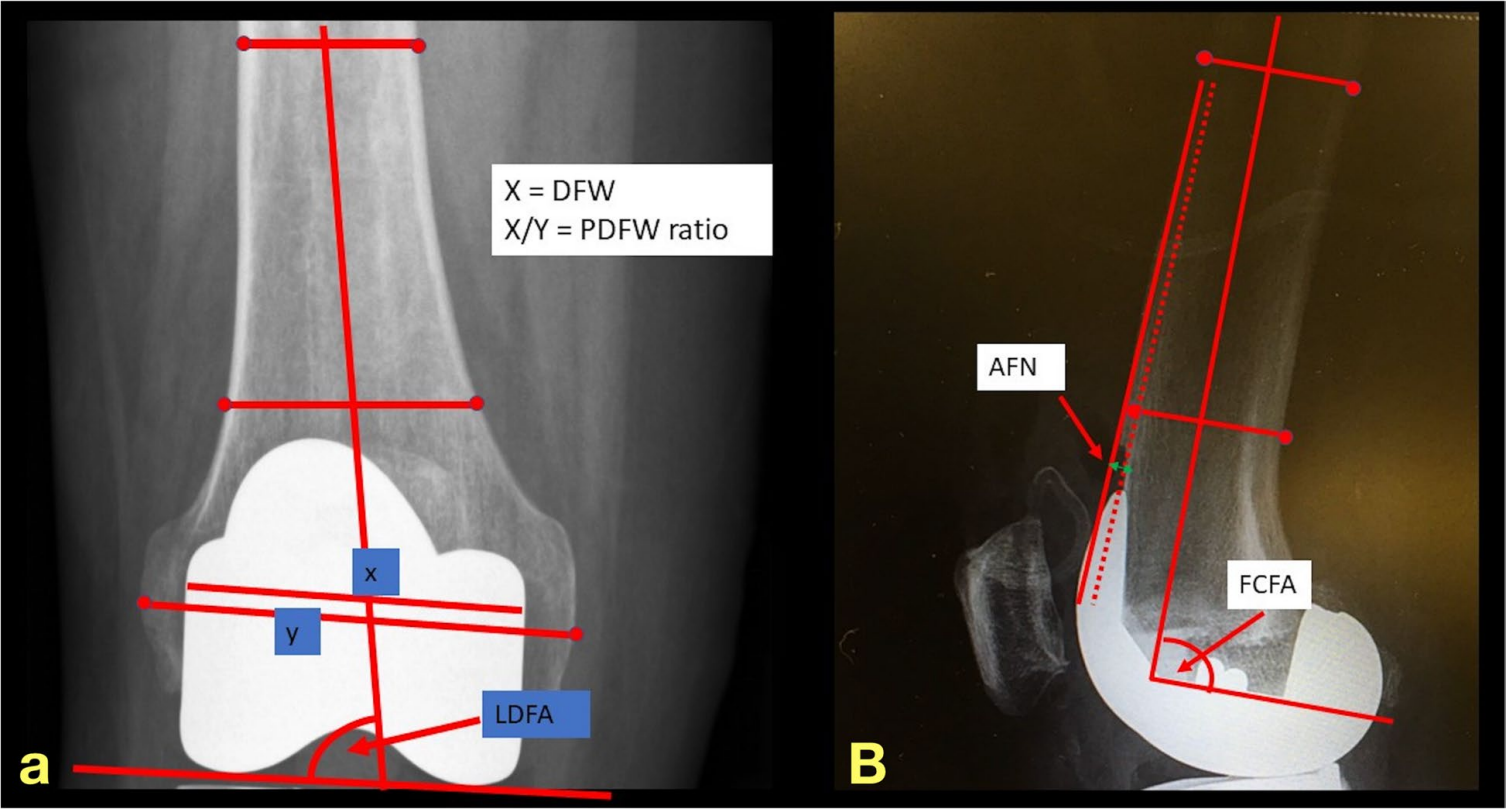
The measurements of knee radiographic outcomes from a) anteroposterior view (anatomical lateral distal femoral angle, LDFA; distal femoral width, DFW; and prosthesis-distal femoral width ratio, PDFW) and b) lateral view (femoral component flexion angle, FCFA; and anterior femoral notching, AFN)
